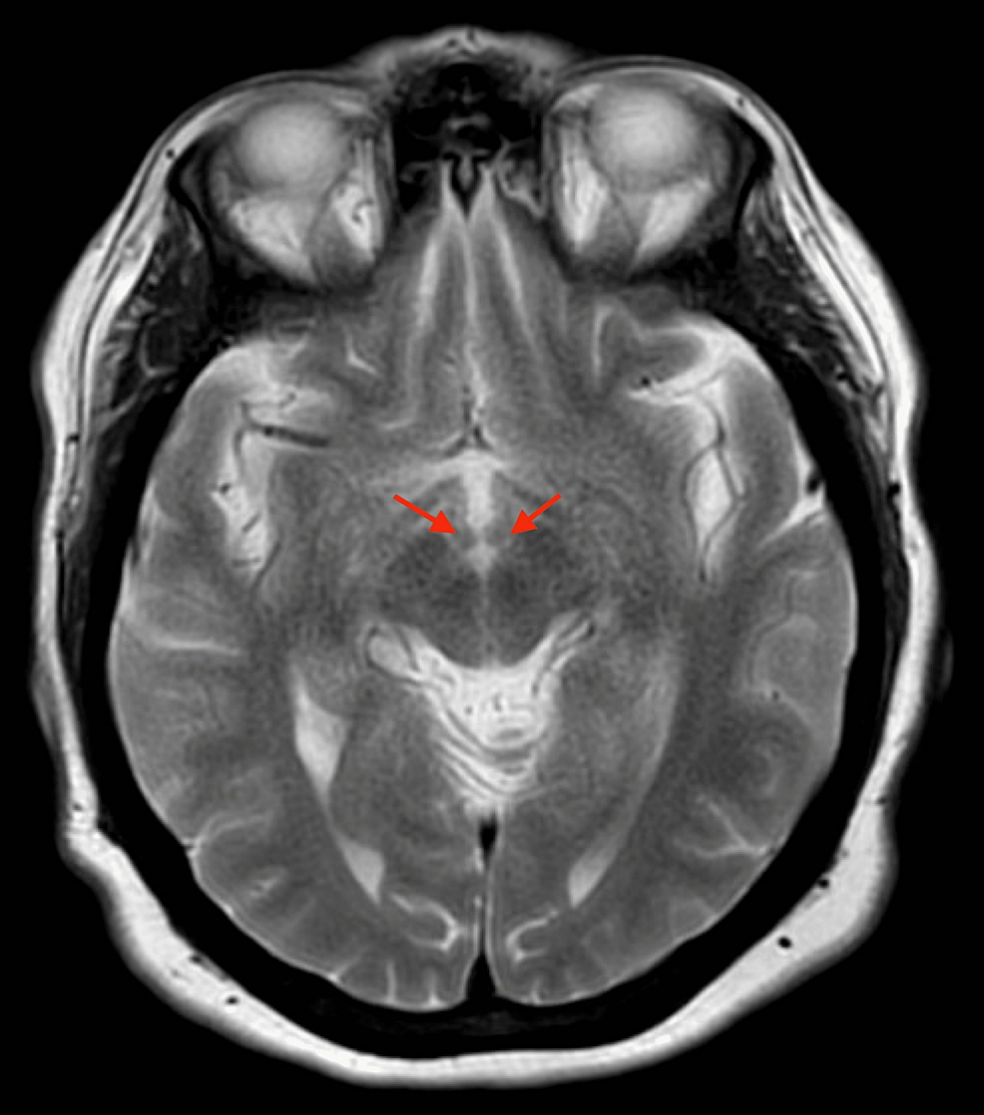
MRI of brain without contrast, T2, axial view. The red arrows are pointing to the bilateral mammillary bodies, which show no hyperintensities. No other abnormal findings were seen on MRI.
